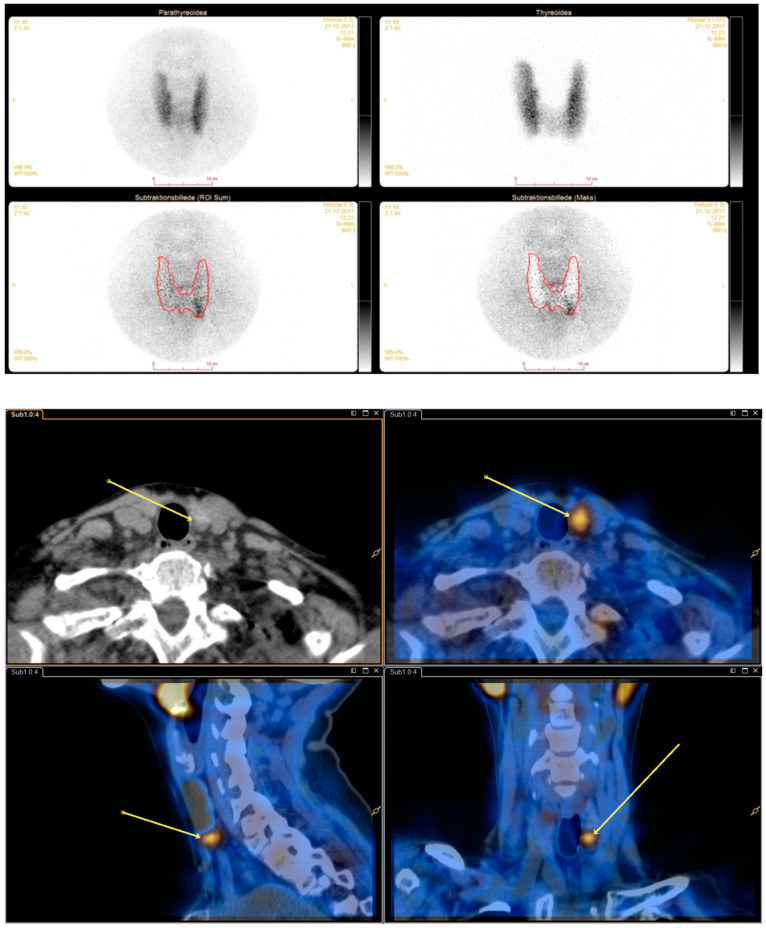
Dual isotope planar pinhole subtraction scintigraphy and dual isotope subtraction single-photon emission computed tomography/computed tomography (SPECT/CT) images. (top image) Upper left: Tc-99m-sestamibi pinhole. Upper right: I-123 pinhole. Middle left and right: Varying degrees of subtraction. (bottom image) Dual isotope subtraction SPECT/CT with and without fusion.
